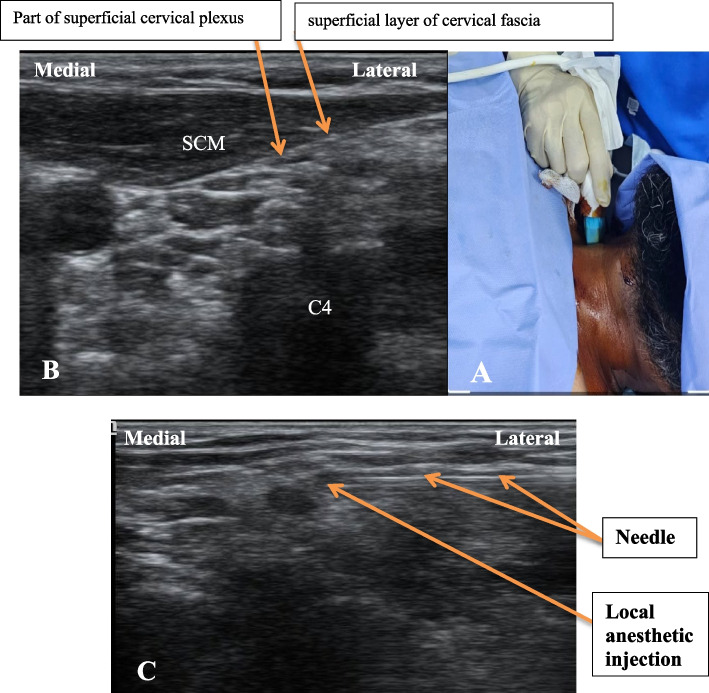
A Site of ultrasound probe. B Sono-anatomy of Superficial cervical block. C Local anesthetic injection
